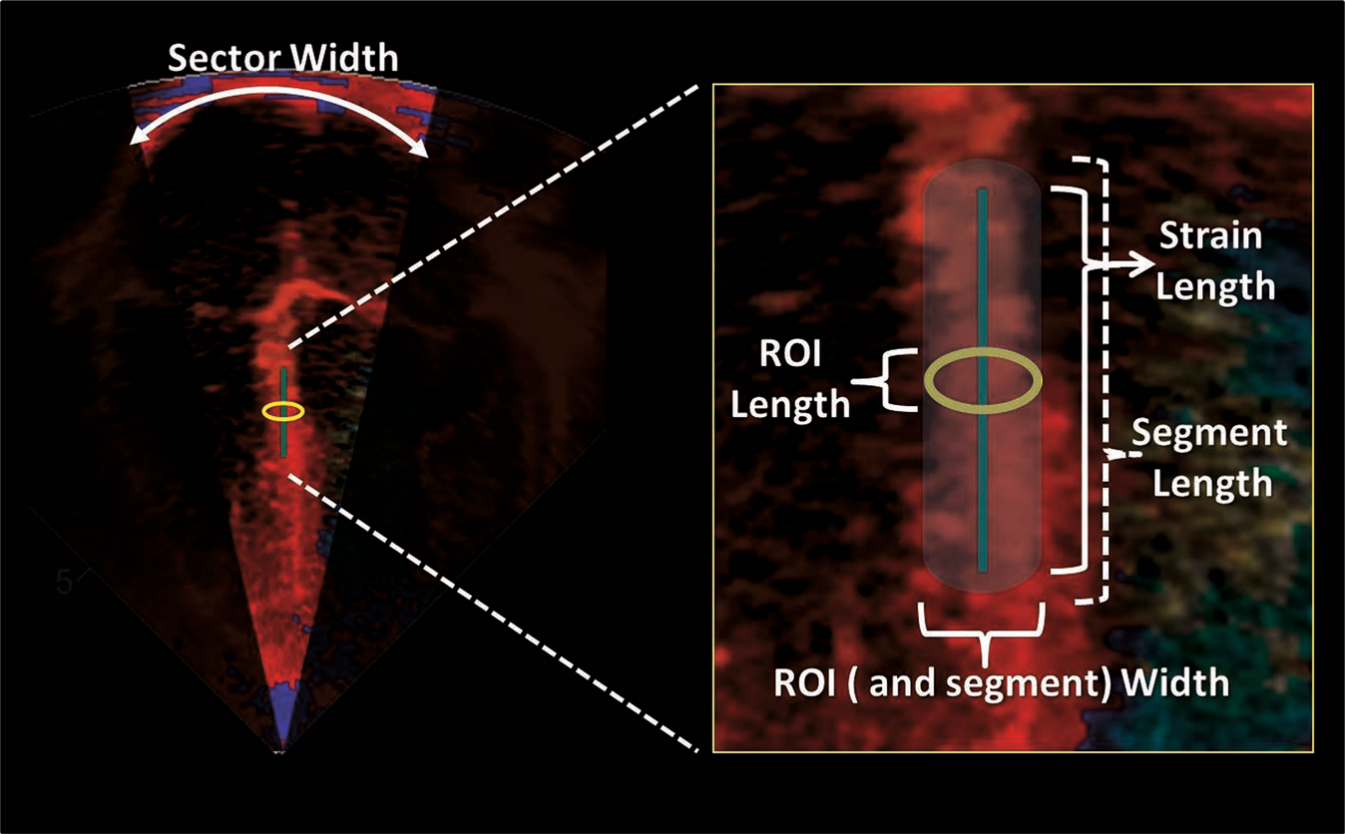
Offline measurement of SR and strain using tissue Doppler. The sector width should be narrowed to increase the frame rate. The basal segment of the wall is usually interrogated to obtain SR and strain values. The ROI dimensions (length and width) are set by the operator. Strain length is also set while ensuring that the borders of the segment are not in contact with artifact or atrial tissue. The ROI can be moved slightly along the wall to obtain a clean and noise-free SR and strain curve (see Fig. 7)
